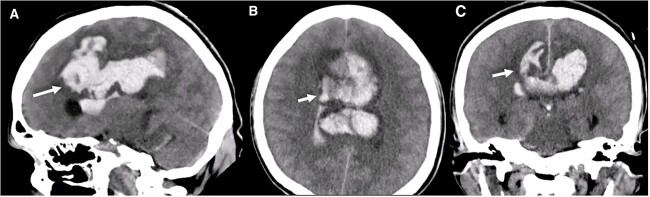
Brain CT without contrast shows a large interhemispheric hematoma, intraventricular hemorrhage, and subarachnoid hemorrhage (white arrows) in (A) Sagittal, (B) Axial, and (C) Coronal views.
